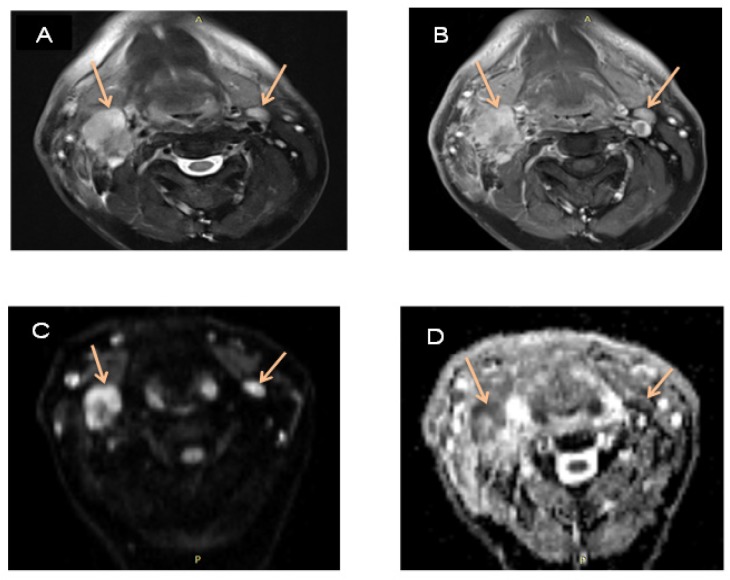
A 45 Year-Old Male with Thyroid and Larynx Cancer. A, T2-W fat sat shows two enlarged right and left neck LNs; B, T1W post contrast shows enhanced LNs; C, DWI shows high signal intensity; D, ADC displays restricted diffusion of LNs on ADC (0.913 × 10^-3^ mm^2^/s)
